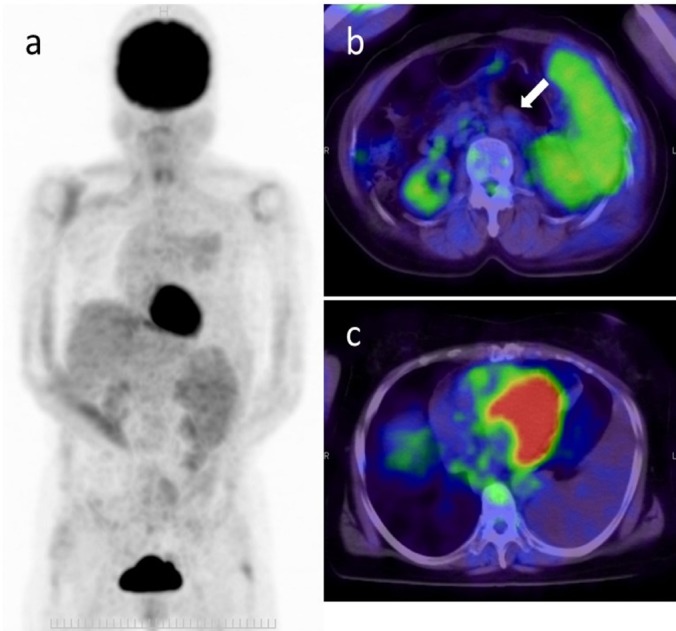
A 59-year-old woman with lymphoma; (a) Maximum intensity projection image and (b, c) fused FDG-PET/CT images are shown. The patient was referred to our hospital for further evaluation and treatment of left pleural effusion, splenomegaly, and paraaortic lymphadenopathy. The level of sIL-2R was within normal limit (378 U/ml). FDG-PET/CT showed splenomegaly with diffusely increased FDG uptake (SUV_max_=3.9) (b) and left pleural effusion (c). Although paraaortic lymphadenopathy was also observed (b; white arrow), the FDG uptake was mild (SUV_max_=1.8). The evaluators commented that this case was compatible with low-grade lymphoma. Confirmed diagnosis obtained by splenectomy was peripheral T cell lymphoma
